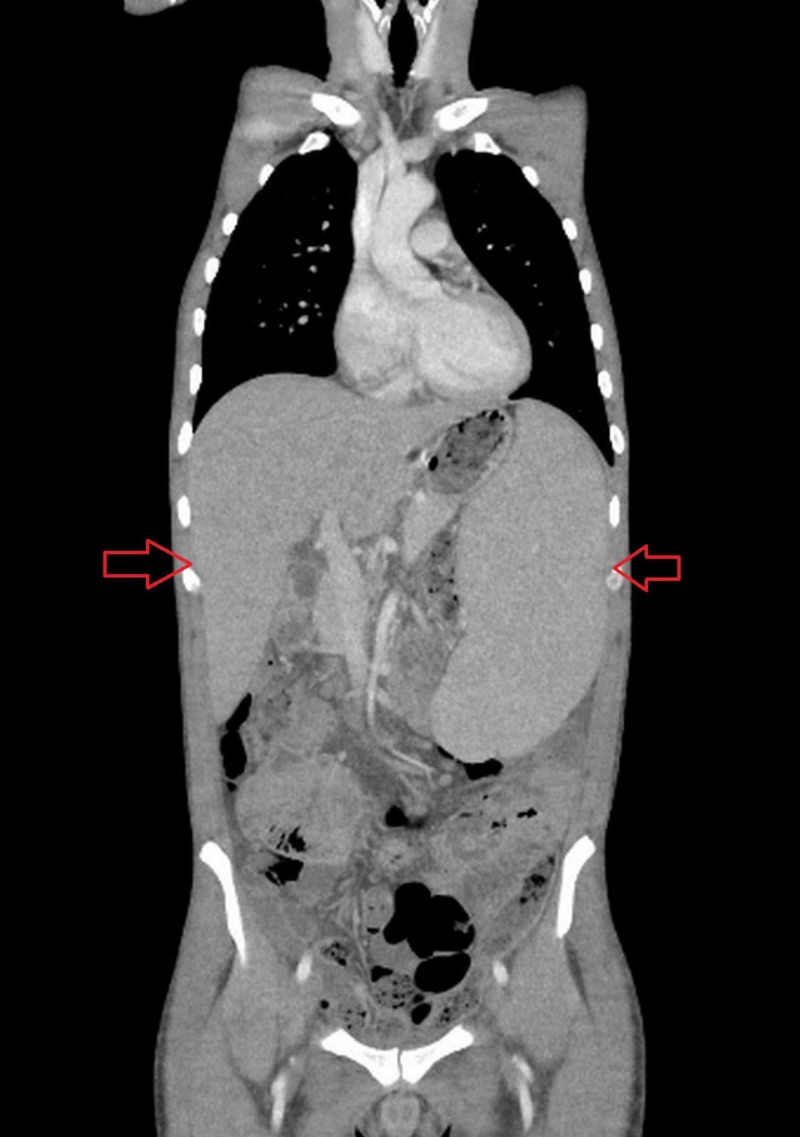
CT CAP showing hepatosplenomegaly (red arrows). CAP: chest, abdomen, and pelvis.
